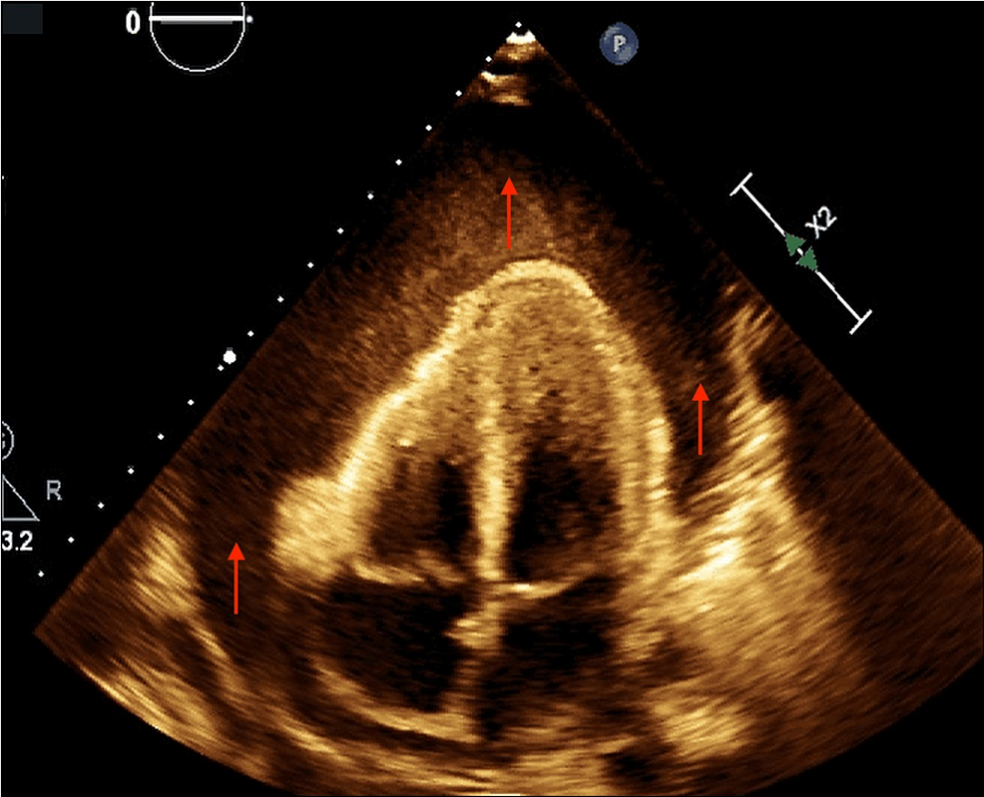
Echocardiogram in an apical four-chamber view shows a large pericardial effusion present circumferentially around the entire heart (red arrows).
